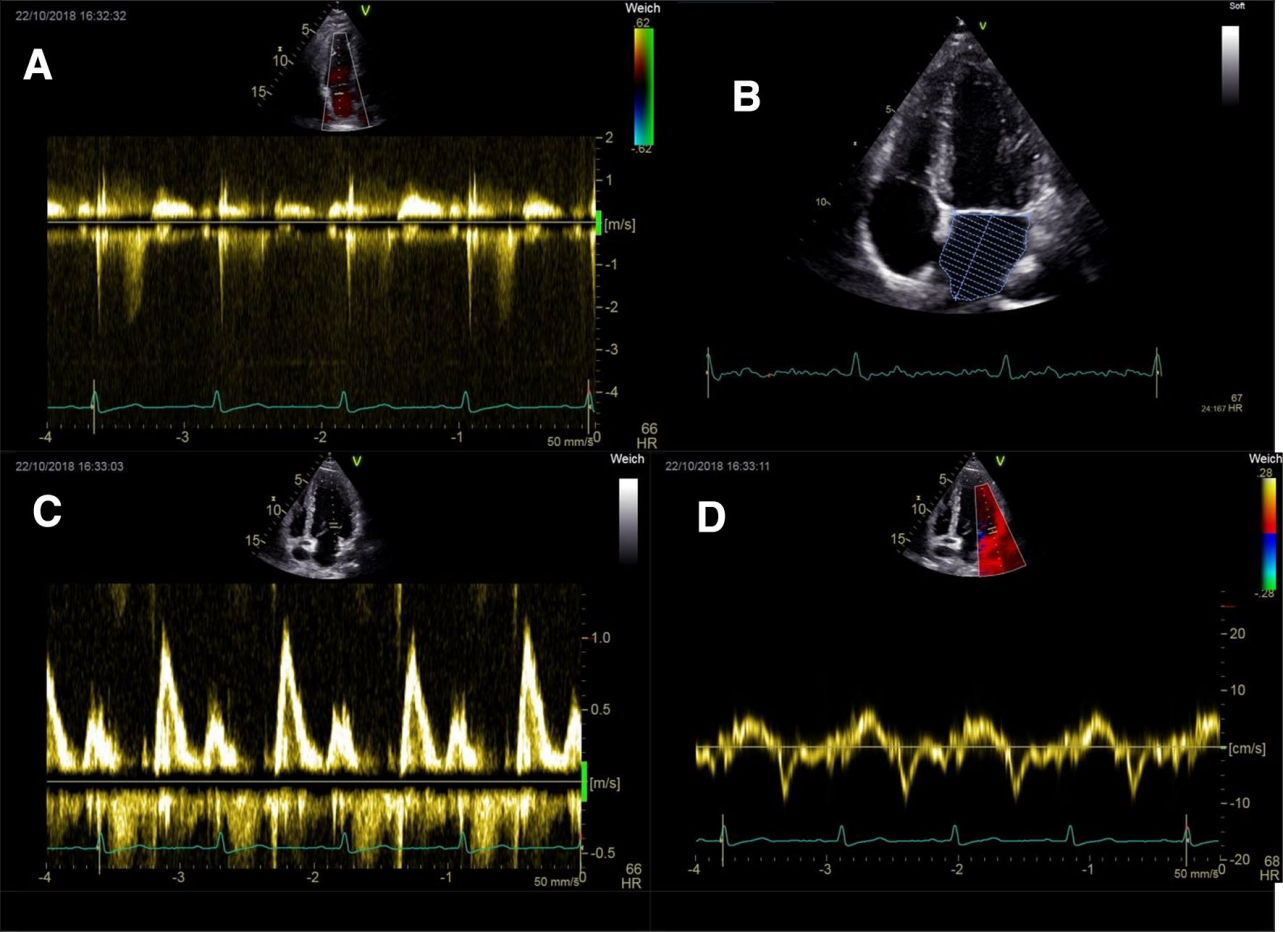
Echocardiographic surrogate parameters allowing for the assessment of left ventricular filling pressures. a Maximal tricuspid regurgitation velocity (TRvmax). b Volume assessment of the left atrium. c PW-Doppler signal of mitral inflow, E-wave and A-wave. d Tissue Doppler imaging of lateral mitral valve annulus, e′. *PW* pulsed wave
